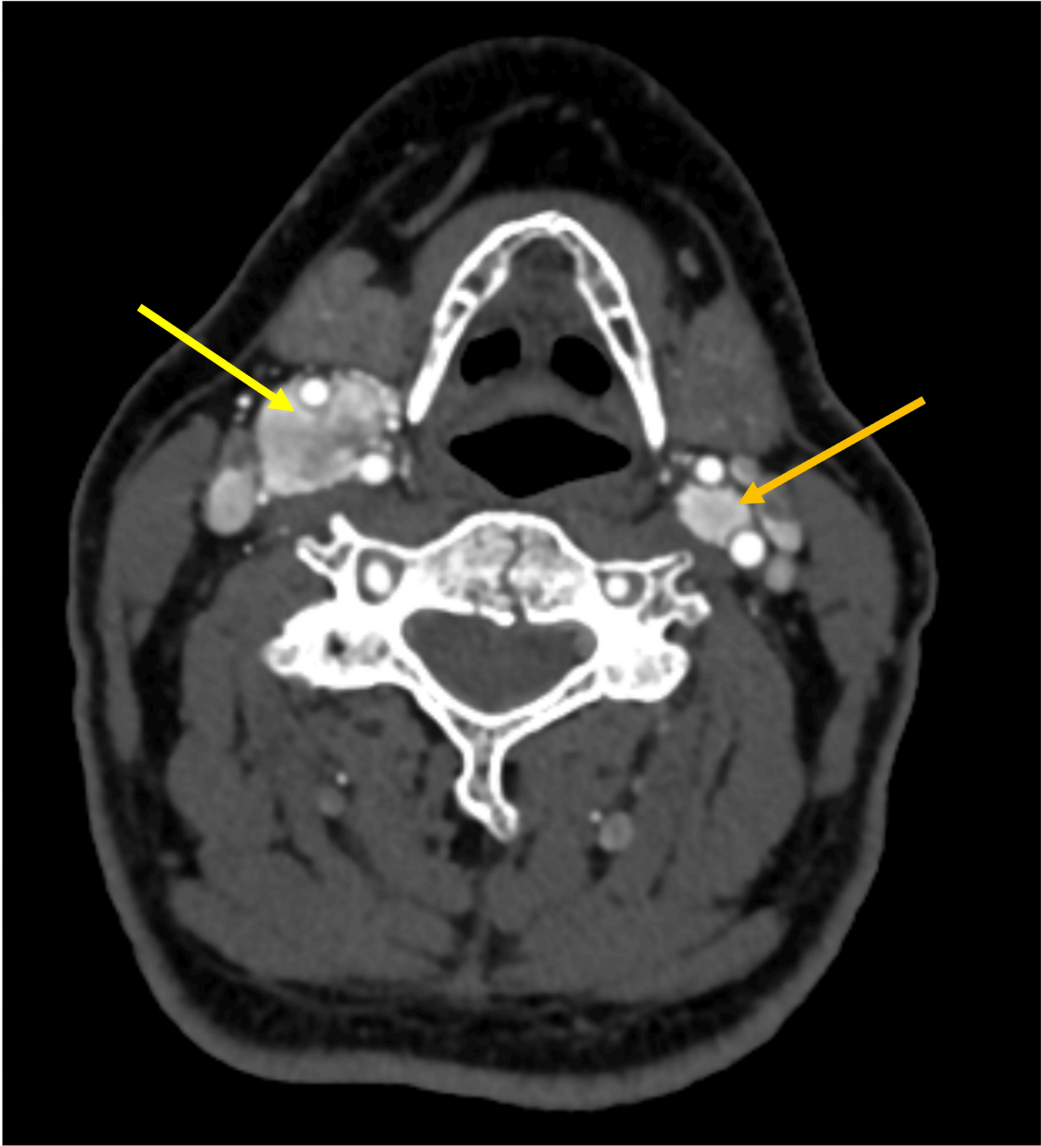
CT angiogram of the neck: Axial section at the level distal to the bifurcation depicting the bilateral carotid body tumors encasing the internal and external carotid arteries on the right (yellow arrow) and abutting the arteries on the left (orange arrow).
